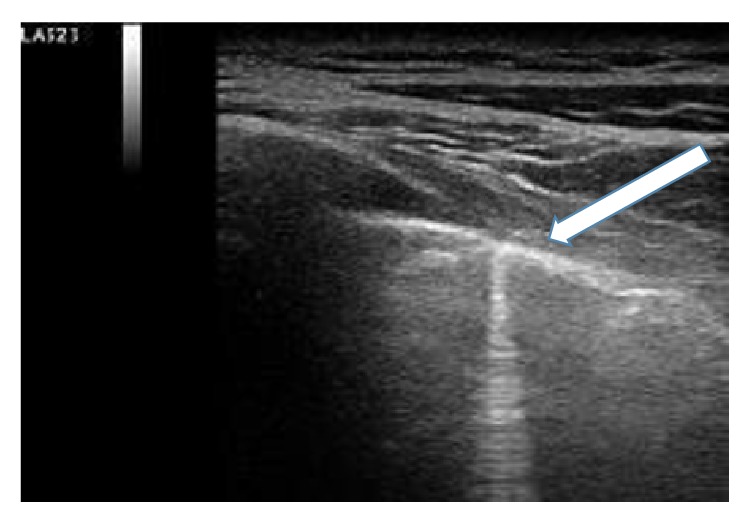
Ultrasound (US) echographic of right lung made in April 2014 before treatment. Presence of Ultrasound Lung Comets (ULCs) at the fifth right posterior intercostal space (arrow).
